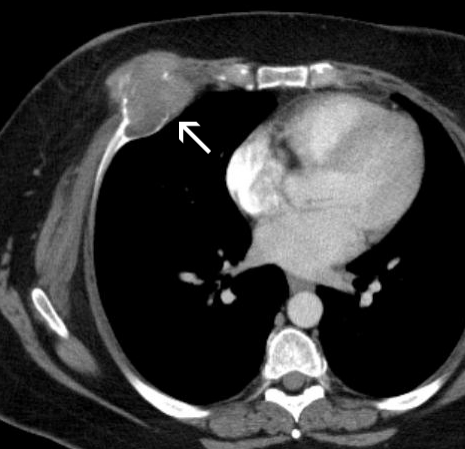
Contrast-enhanced chest CT demonstrates a rounded anterior chest wall mass with osteolysis of the right fifth rib and a large soft tissue component.
